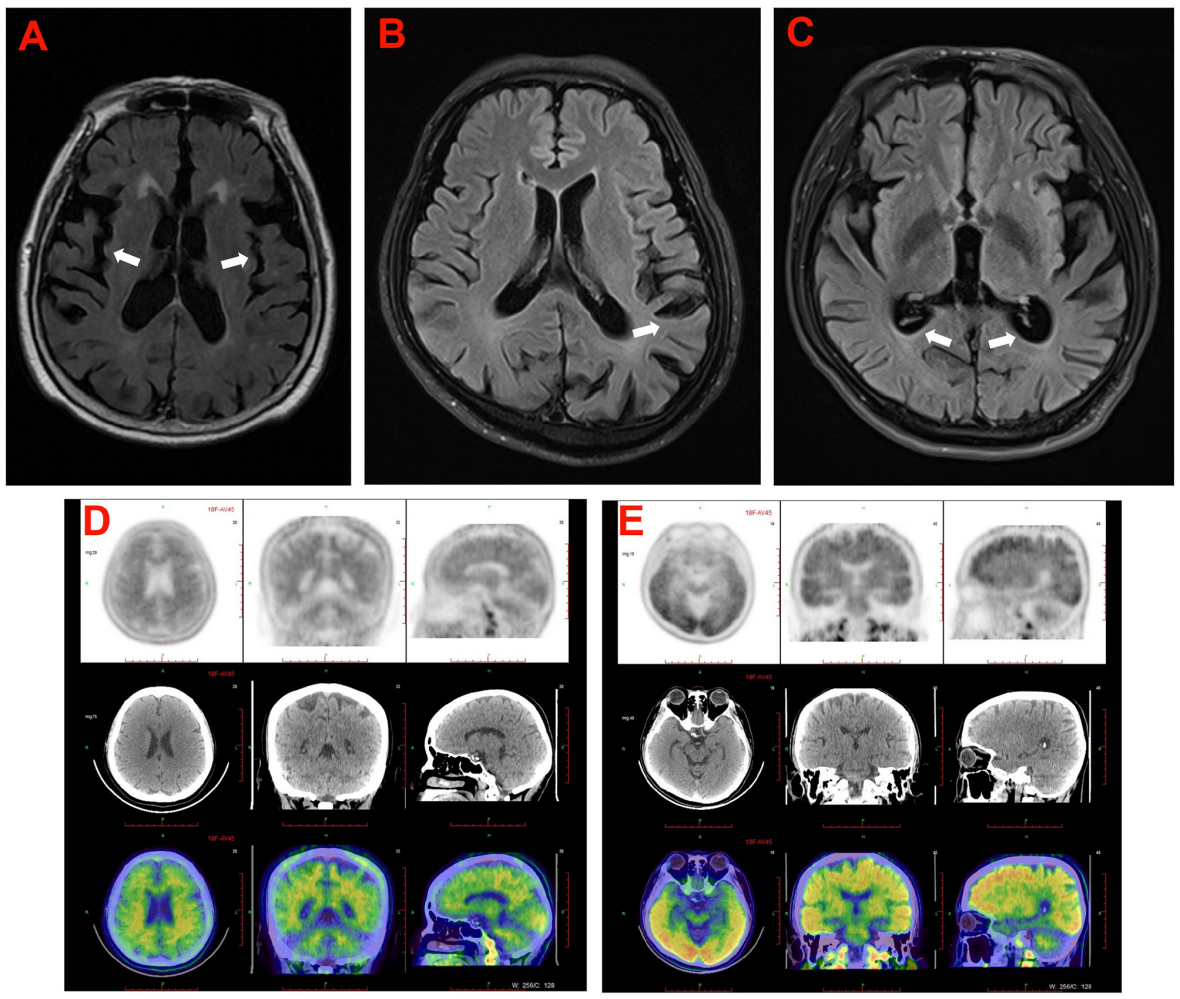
The cranial neuroimaging of the probands in the five families. (A) The T2 weighed image showed atrophy of cerebral cortex (arrowhead) and mild ventriculomegaly with periventricular signal abnormality in proband (II-3) in family 5. (B) The T2 FLAIR image showed atrophy of cerebral cortex (arrowhead) predominantly in temporoparietal lobe of the proband (II-4) in family 4. (C) The T2 FLAIR image showed atrophy of cerebral cortex (arrowhead) and mild ventriculomegaly in proband (III-3) of family 2. (D, E) 18F-AV45 brain images of the proband (II-3) in family 1 (D), and the proband (II-2) in family 3 (E).
